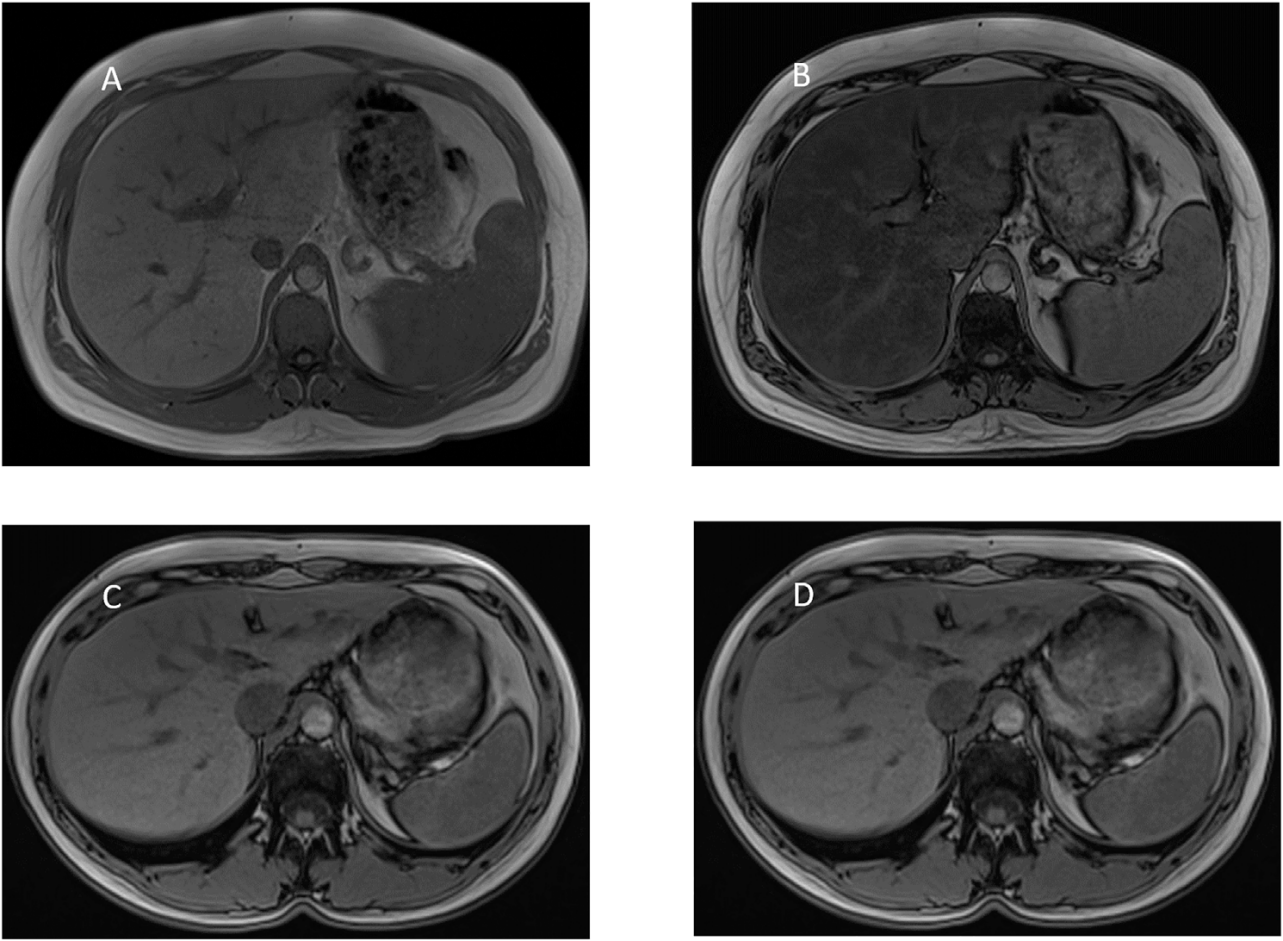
MRI-imaging features of liver steatosis. Caption: MRI proton chemical shift imaging with (A) in-phase T1-weighted gradient-echo image showing normal signal intensity of the hepatic parenchyma and (B) opposed-phase T1-weighted gradient-echo image showing a marked drop in signal intensity of the hepatic parenchyma, indicating diffuse hepatic steatosis. In contrast, MRI proton chemical shift imaging with (C) in-phase and (D) opposed-phase T1-weighted gradient-echo image showing normal signal intensity of the hepatic parenchyma indicating the absence of steatosis. In GlyH, as described by Saikusa et al., there is no drop in intensity either between in- and out-of-phase images.
